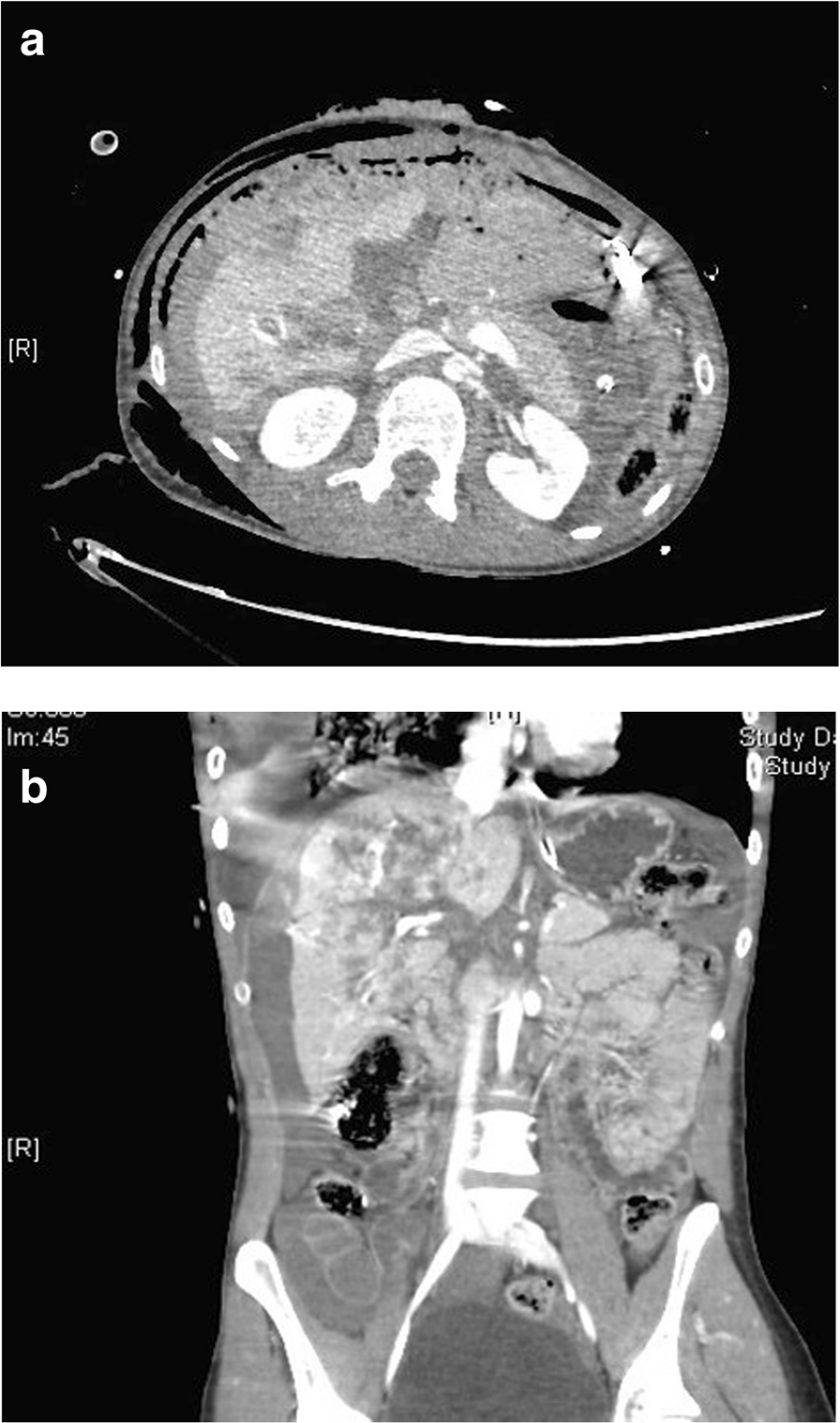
a b CT immages of Grade V liver injury
